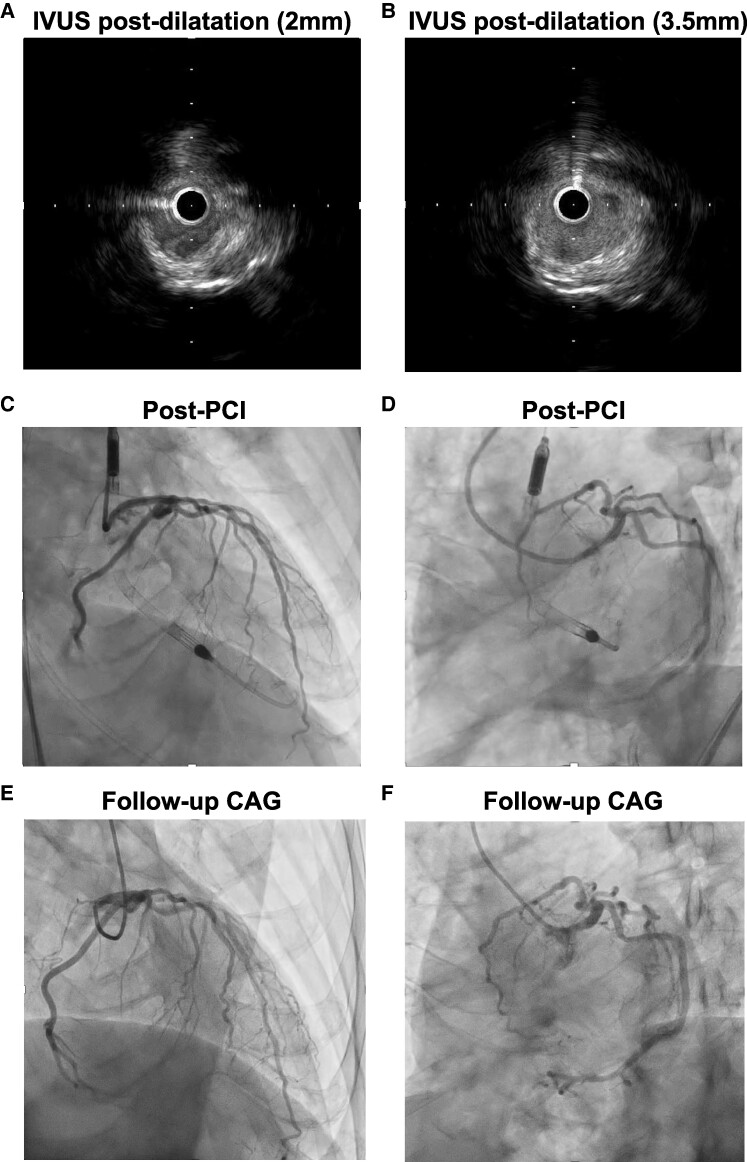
(*A*) Intravascular ultrasound after pre-dilatation with a non-compliant balloon reveals the calcified plaque (minimum lumen area of 3.1 mm^2^). (*B*) IVUS after pre-dilatation with a cutting balloon reveals satisfactory dilatation (MLA of 6.1 mm^2^). (*C*) Coronary angiography post–percutaneous coronary intervention shows a satisfactory dilatation result (right anterior oblique cranial view). (*D*) Coronary angiography post–percutaneous coronary intervention (left anterior oblique caudal view). (*E*) Follow-up coronary angiography on hospital day 9 revealed no restenosis (right anterior oblique cranial view). (*F*) Follow-up coronary angiography on hospital day 9 (left anterior oblique caudal view).
